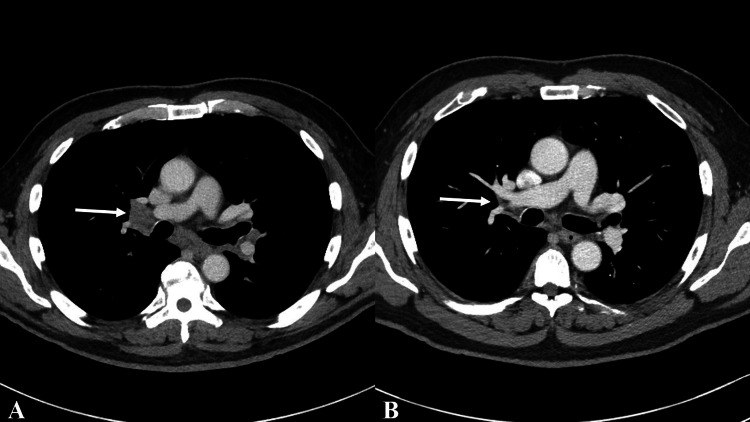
CT images showing suprahilar (arrow) and subcarinal lymphadenopathy (A), with subsequent improvement six weeks after steroid taper (B).
